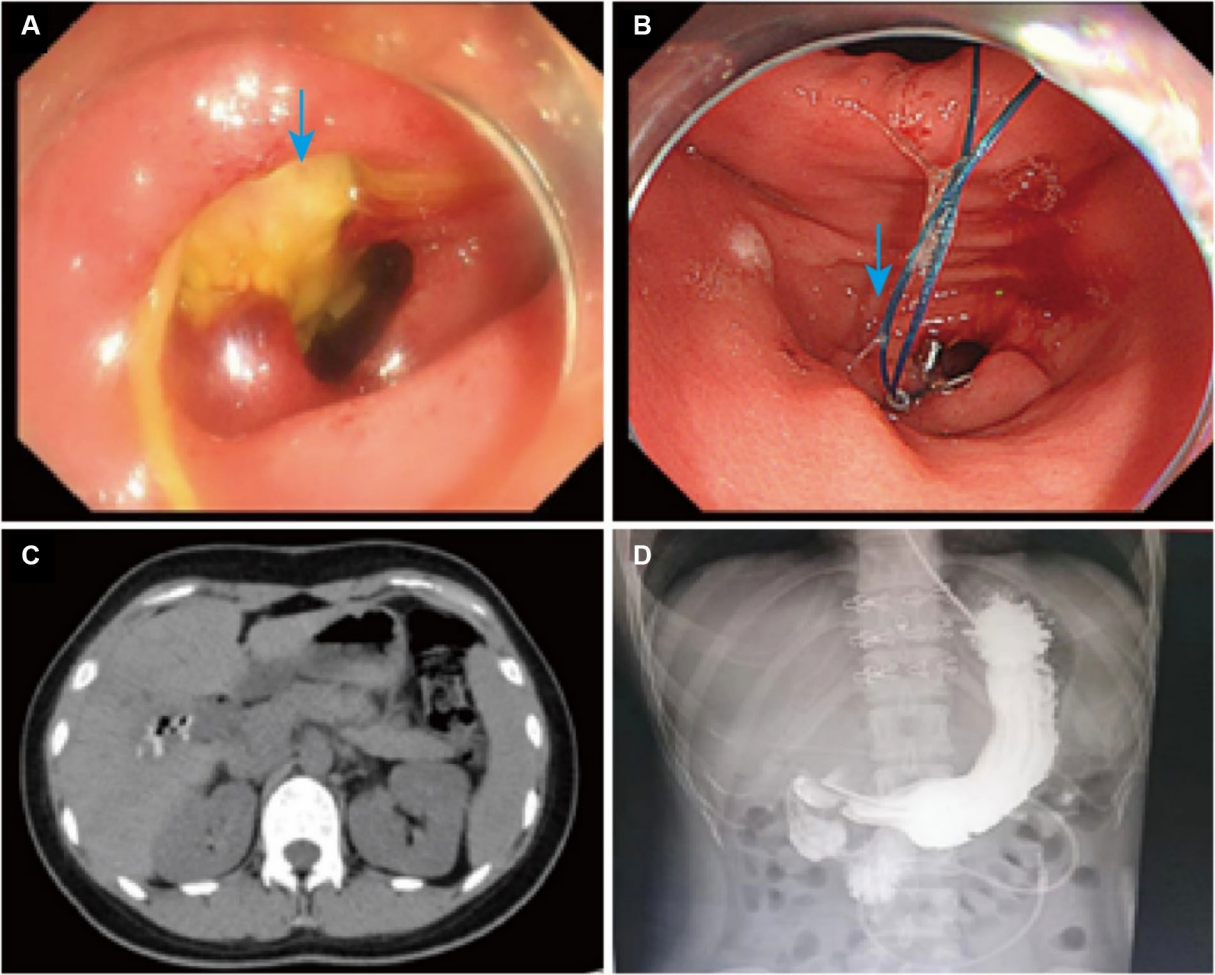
Changes before and after treatment. (A) Physical picture before treatment. The blue arrow points to the duodenal fistula. (B) Physical picture after treatment. The blue arrow points to the post-surgical trauma. (C) The post-treatment CT image of abdomen. (D) The upper gastrointestinal imaging result after treatment.
